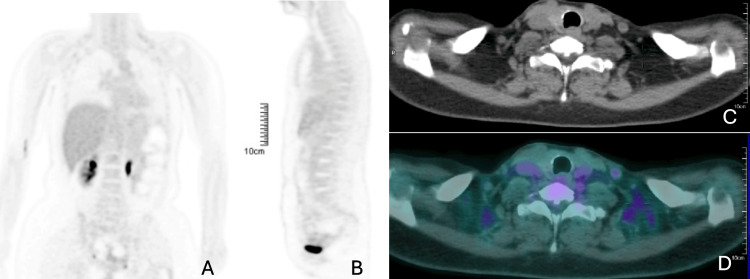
Whole-body PET/CT at the time of neurological symptom onset showing no evidence of metabolically active disease (February 2025) (A) Coronal FDG-PET image demonstrating physiological tracer distribution without abnormal hypermetabolic foci in the thorax, abdomen, or pelvis. (B) Sagittal FDG-PET image likewise showing absence of pathological FDG uptake. (C) Corresponding axial CT image at the cervicothoracic level showing no structural abnormalities or suspicious soft-tissue lesions. (D) Axial fused PET/CT image of the same level, confirming the absence of abnormal FDG uptake and supporting the lack of metabolically active disease. PET/CT: positron emission tomography/computed tomography; FDG: fluorodeoxyglucose
